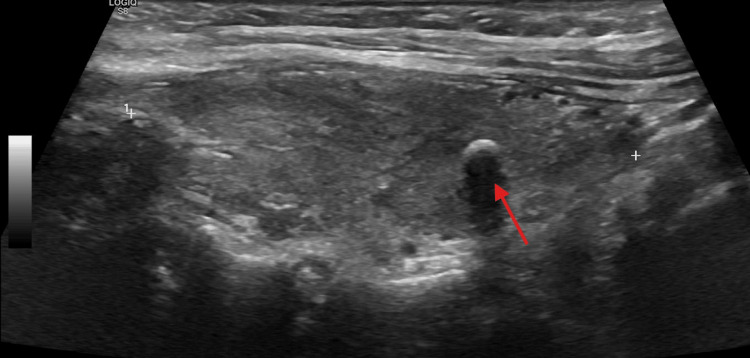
Thyroid ultrasound findings Sagittal ultrasound image showing a well-circumscribed nodule in the right thyroid lobe.
